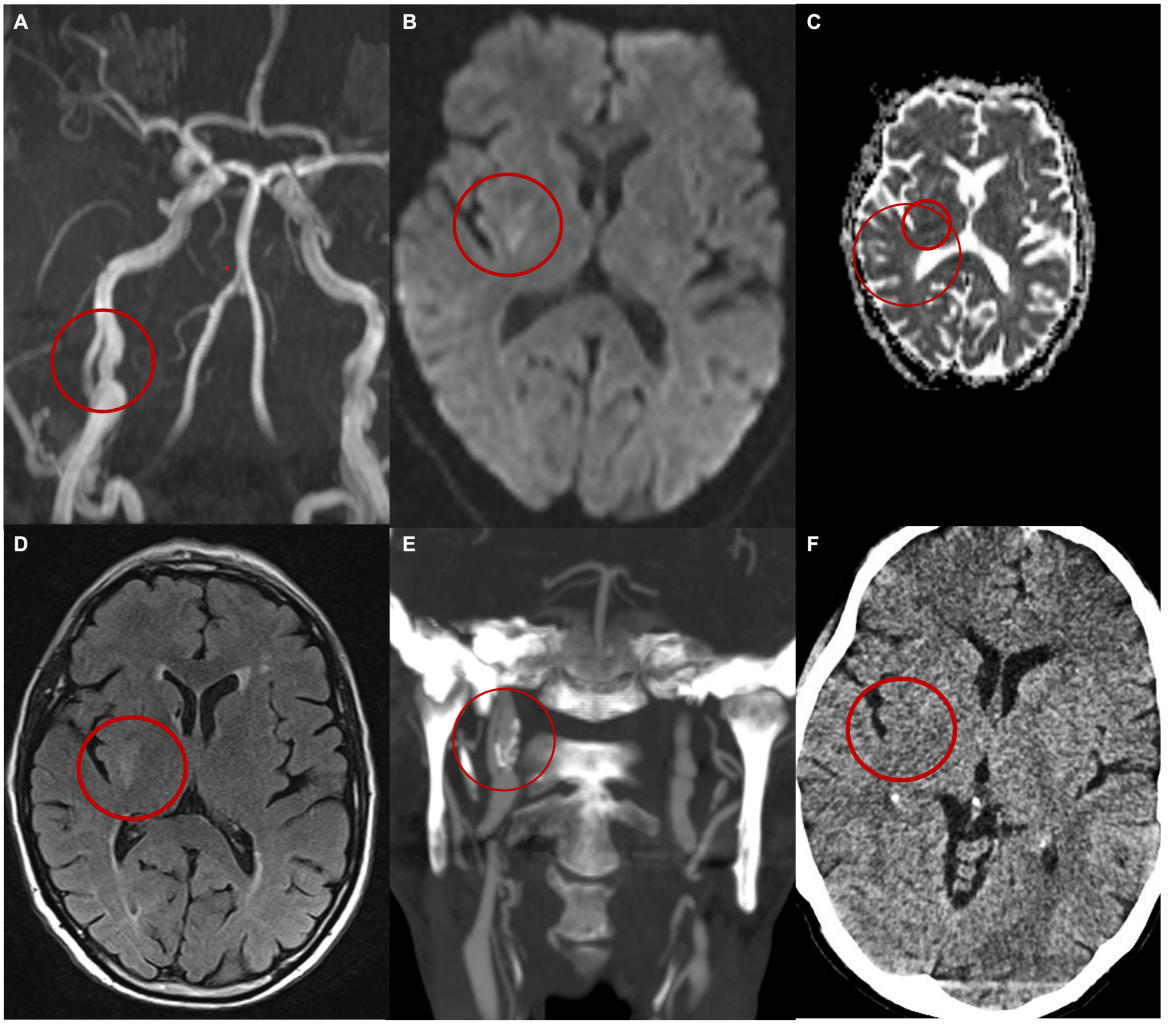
Patient’s imaging. (A) MIP MR angiography with gadolinium images reveals a dissection of the right internal carotid artery characterized by the presence of a double lumen and an intimal flap. (B) MRi DWI shows right capsular hyperintensity compatible with an acute ischaemic lesion of the right capsule. (C) ADC shows restriction of the diffusivity. (D) MRi FLAIR. Images at the 6-month follow-up (E) shows on the CT angiography the persistence of a double lumen and the presence of an aneurysmal dilation in the true lumen (E). (F) CT brain at 6 months.
